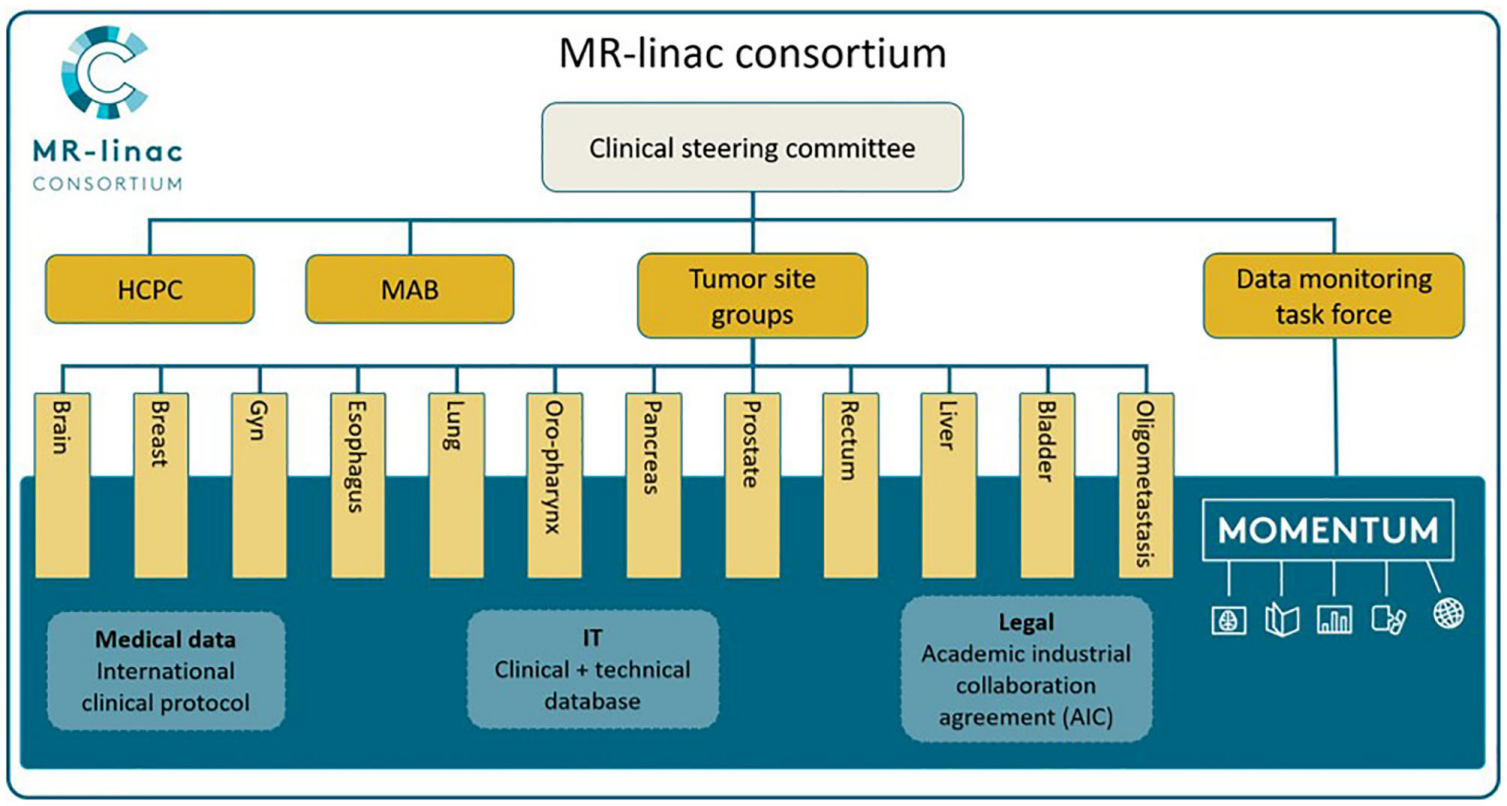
The MR-linac Consortium and its organizational structure. Adapted from “The MRI-Linear Accelerator Consortium: Evidence-Based Clinical Introduction of an Innovation in Radiation Oncology Connecting Researchers, Methodology, Data Collection, Quality Assurance, and Technical Development” by Kerkmeijer et al. (15), Frontiers in Oncology, 6, P. 1–6. HCPC, Health Care Policy Committee; MAB, Methodology Advisory Board.
